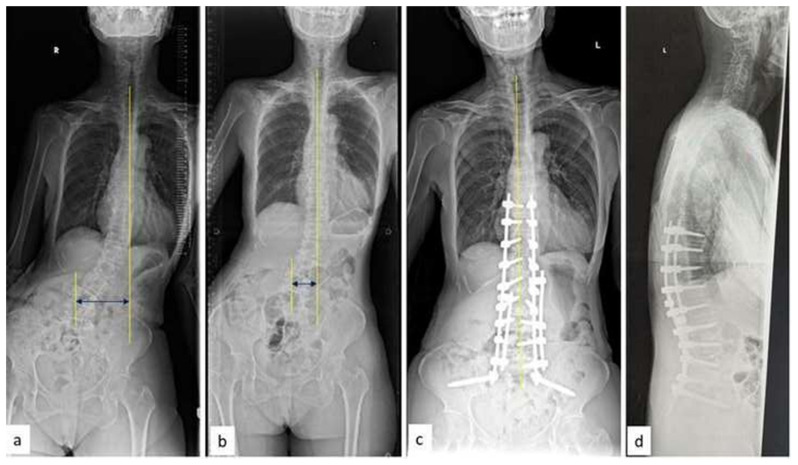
(a) Pre-operative Whole Spine Antero-Posterior radiograph showing lumbar scoliosis with significant imbalance in coronal axis (b) Whole Spine AP radiographs after stage I OLIF surgery showing about 70 % improvement in coronal balance (c–d) Three years follow-up radiographs (after T10-Pelvis posterior stabilization) showing a well-balanced spine in sagittal and coronal axes.
